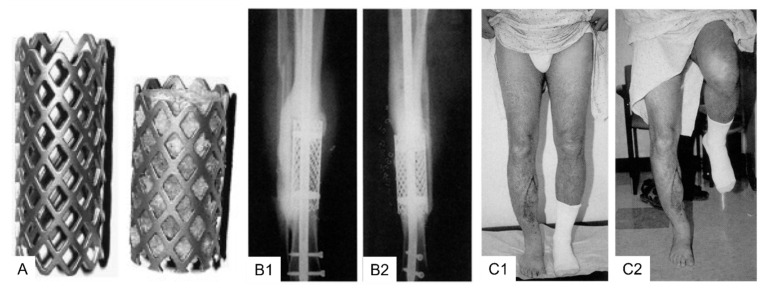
First case report from 1999 by Cobos et al. [123] on the treatment of a patient (34-year-old) with post-traumatic segmental defect of the tibia (8.5 cm) with a cylindrical (spinal) titanium mesh cage in combination with cancellous bone allograft and demineralized bone matrix putty (Grafton) stabilized with an intramedullary nail. The titanium mesh cage was trimmed to 9 cm (diameter 2.2 cm) to fit the defect size and packed with allograft prior to implantation (A). Posterolateral callus consolidation around the cage on X-ray (views: (B1): anterior–posterior; (B2): lateral) 12 months post-reconstruction. Standing patient (C1) and standing on the operated limb (C2) at one-year follow-up. Adapted from Ref. [22], reproduced with permission from John Wiley & Sons, Inc.
